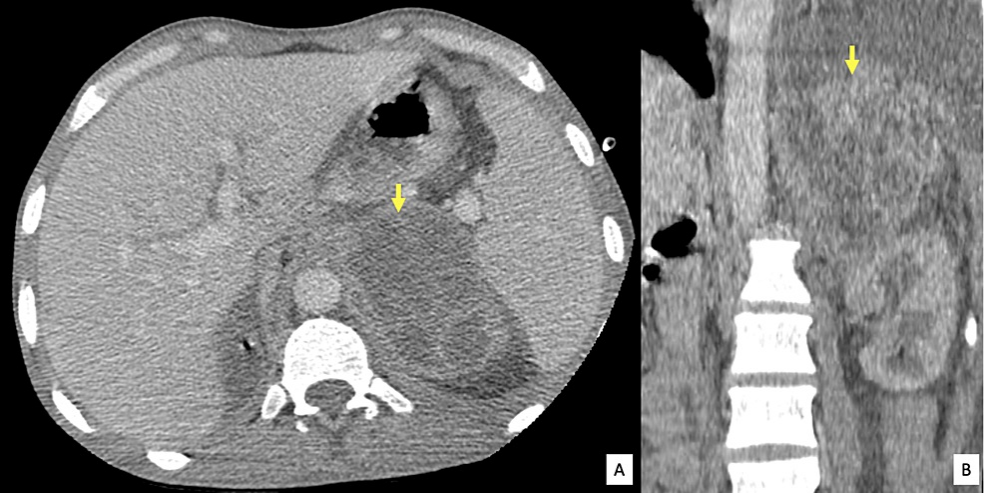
Axial (A) and coronal (B) CT images Left adrenal mass with lobulated margins and heterogenous enhancement with no calcifications and no invasion of the adjacent upper pole of the kidney, medial aspect of the spleen, or diaphragm (arrows).
